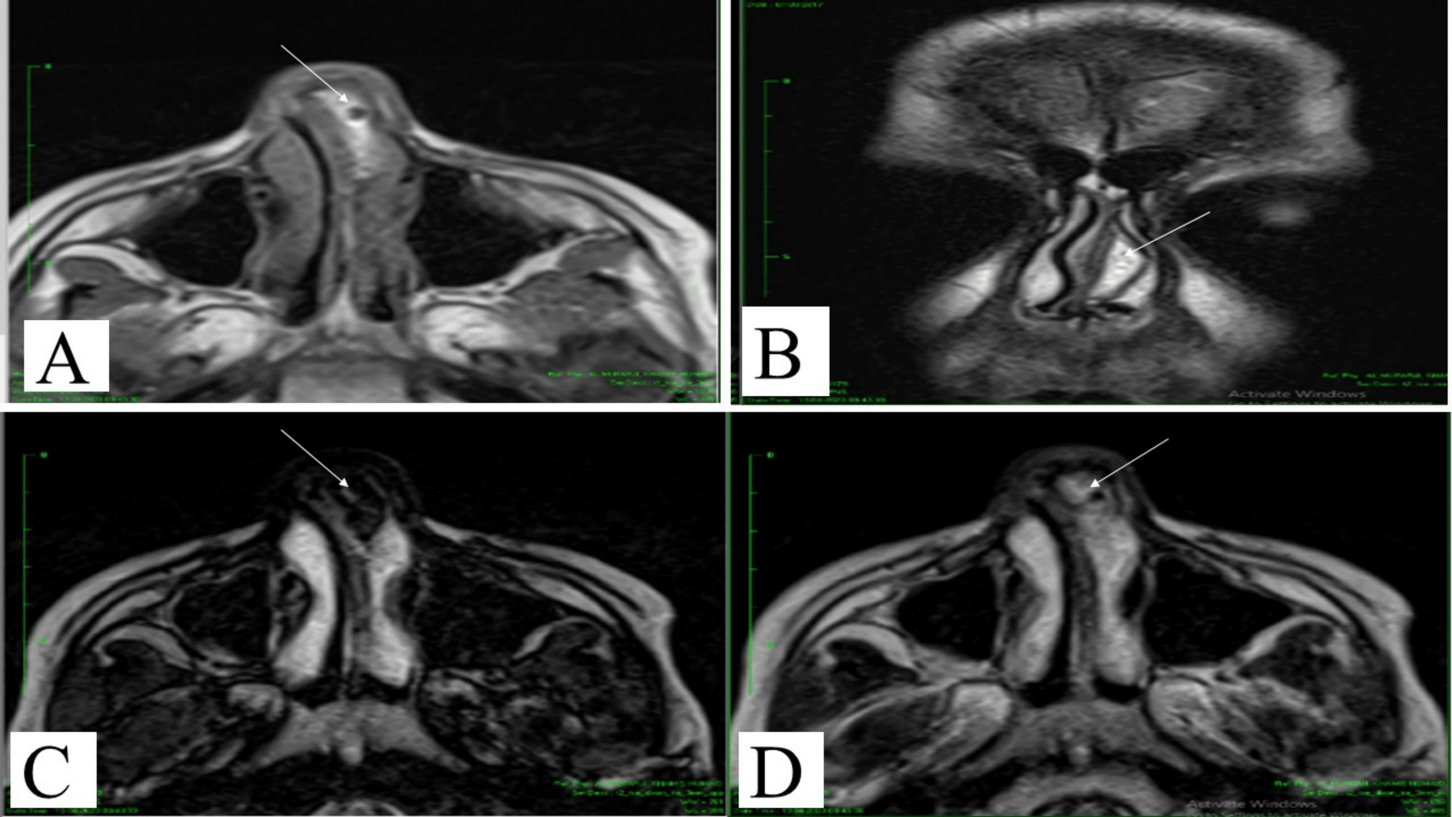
MRI of the septal lipoma A) Axial T1 MRI image showing homogenous high signal intensity at the left side of the nasal septum. B) Coronal T2 MRI image showing intermediate high signal intensity at the left side of the nasal septum. C, D) Dixon MRI (C out, D in) sequences. C) shows low signal intensity at the site of the lesion, representing fat suppression. D) shows high signal intensity at the site of the lesion, representing fat. MRI features are consistent with lipoma.
